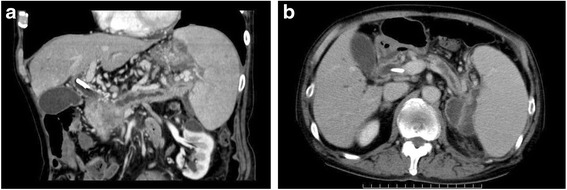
Abdominal CT showing a low-density mass, measuring 40 × 30 mm, in the pancreatic head associated with a marked dilatation of the bile duct and distal pancreatic duct (a). There was also an irregular-shaped cystic lesion spreading from the pancreatic tail to the splenic hilum, suggestive of a pancreatic pseudocyst associated with severe obstructive pancreatitis (b)
